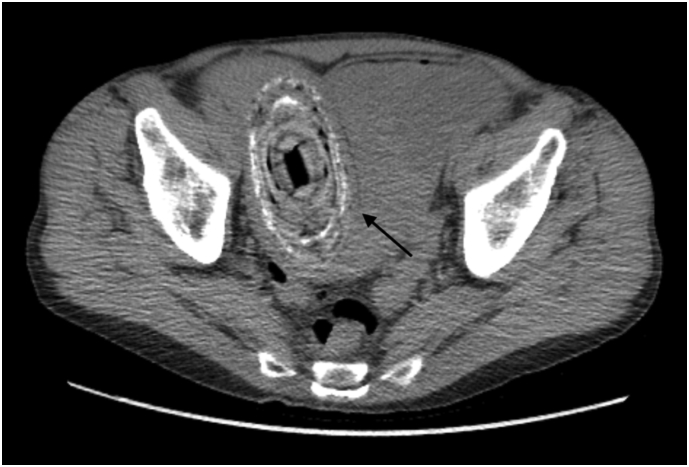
Axial CT scan - foreign body in small bowel (arrow).
